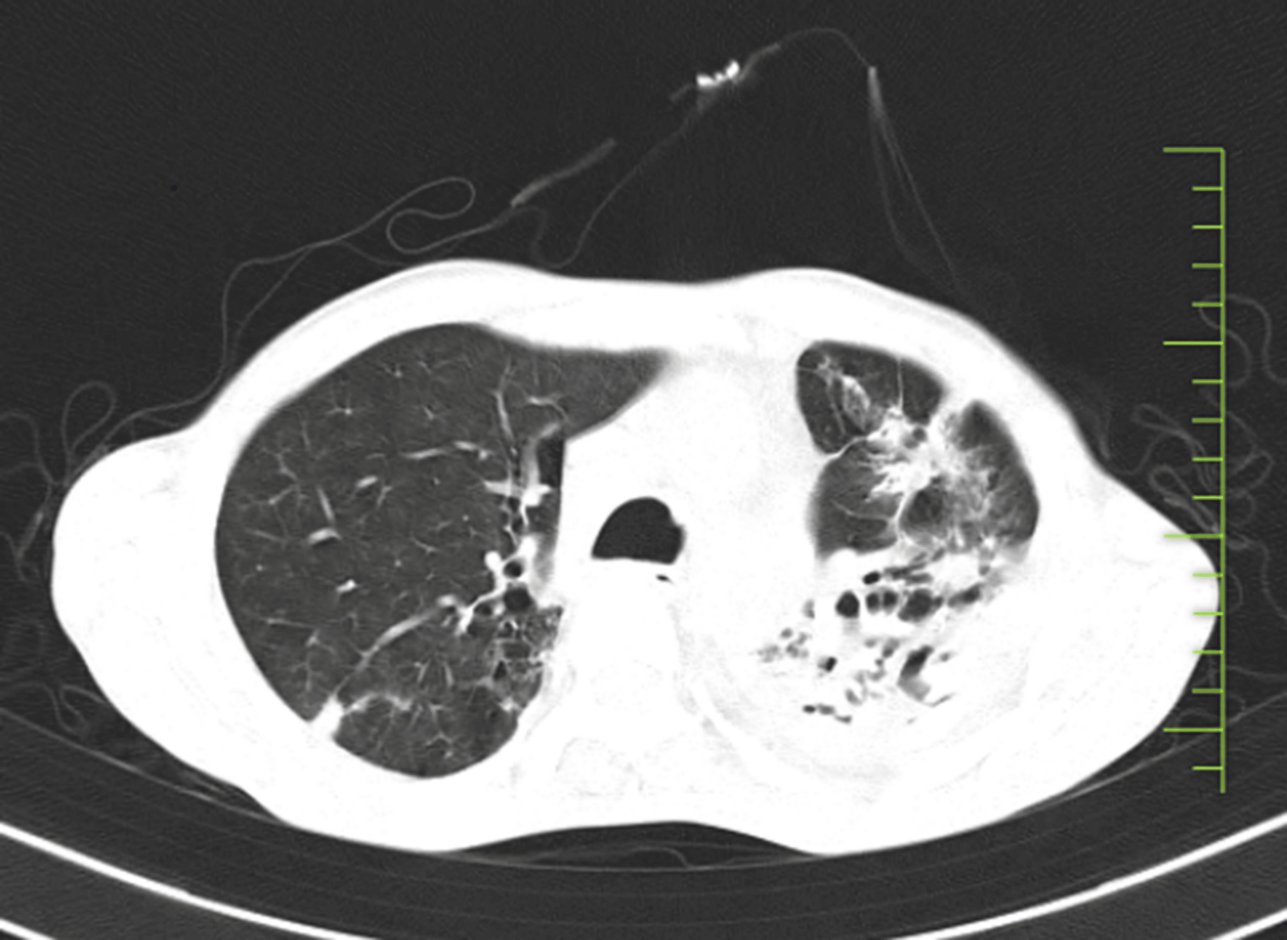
High-resolution computed tomography (HRCT) thorax suggestive of old tuberculosis with active infective etiology
